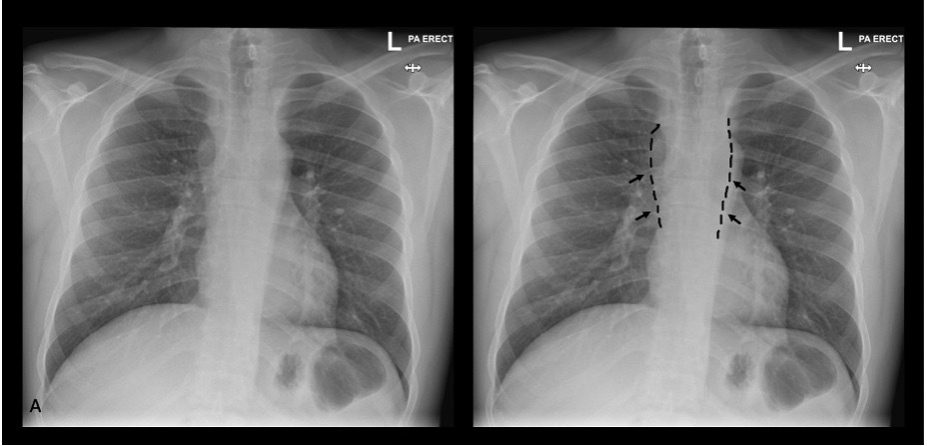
Image A and annotated image B are posteroanterior erect chest radiographs showing soft tissue density bulging along the right and left paravertebral lines (delineated on image B, note dotted lines and black arrows) representing underlying paraspinal abscesses seen on MRI.
